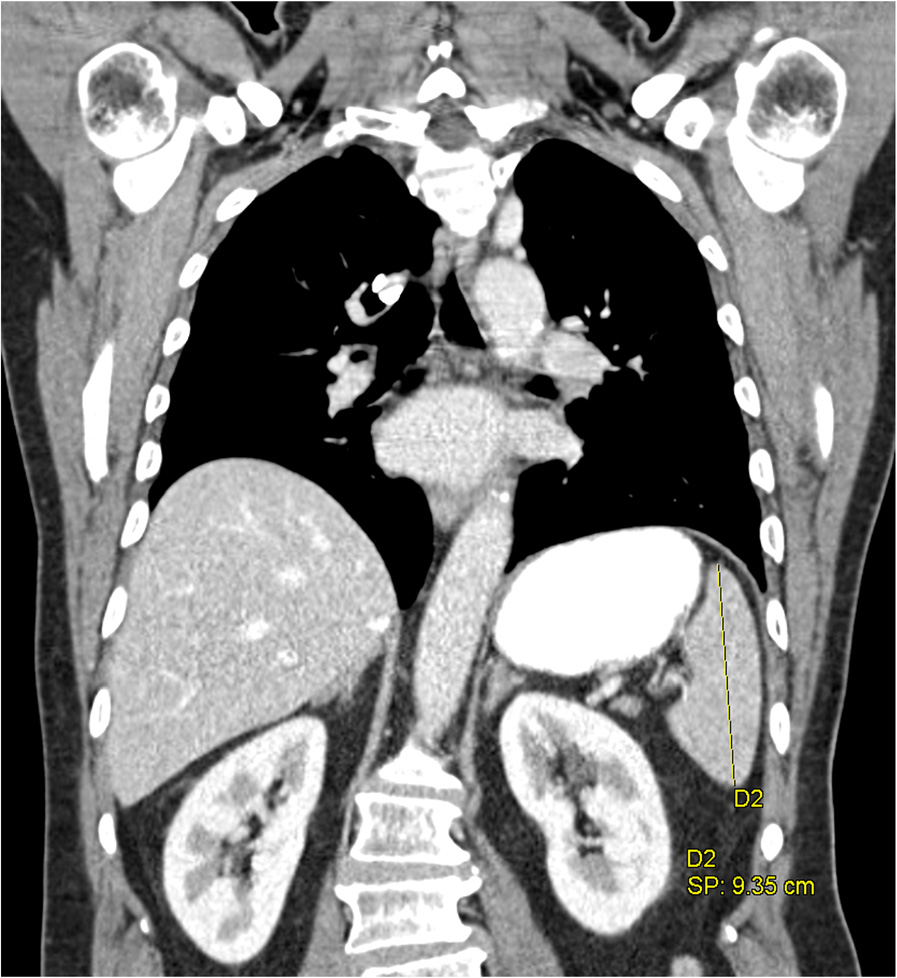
Craniocaudal extension of the spleen. A coronal slice of a contrast-enhanced computed tomography examination of the upper thorax/upper abdomen. The height of the spleen is measured in parallel to the craniocaudal axis
